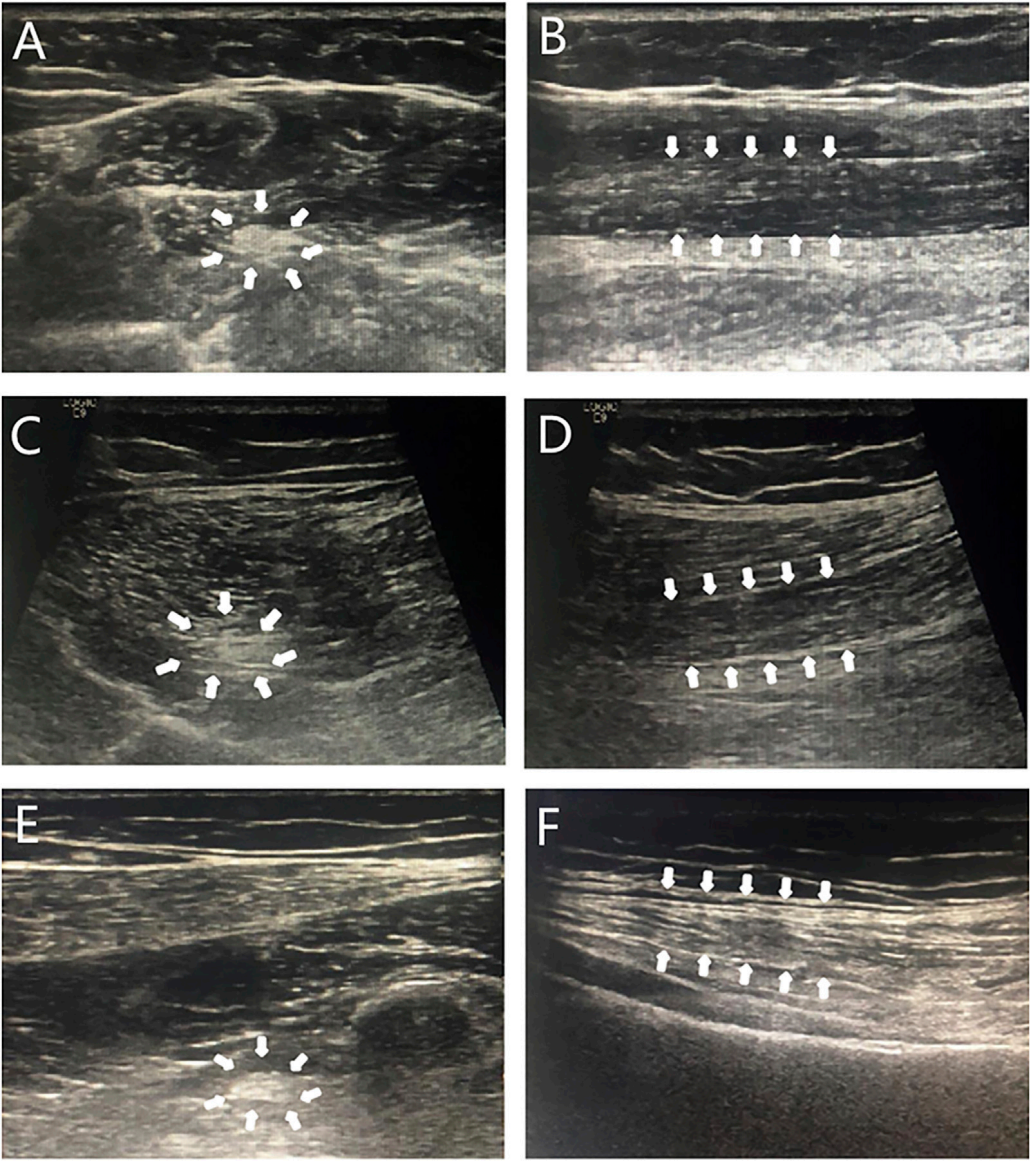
Sonogram of neurosonography. Note: Transverse sections (A,C,E): punctate hyperechoic; longitudinal sections (B,D,F): diffuse hyperechoic, less clear texture. A and B: median nerve; C and D: tibial nerve; E and F: peroneal nerve; arrows: the area shown was an abnormal signal on neurosonography.
